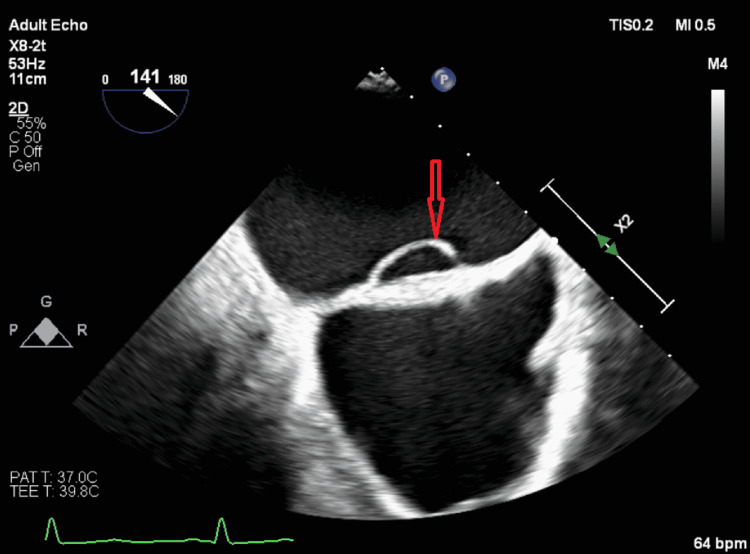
TEE mid-esophageal view at 141 degrees showing a LASP (red arrow) TEE: transesophageal echocardiogram; LASP: left atrial septal pouch
